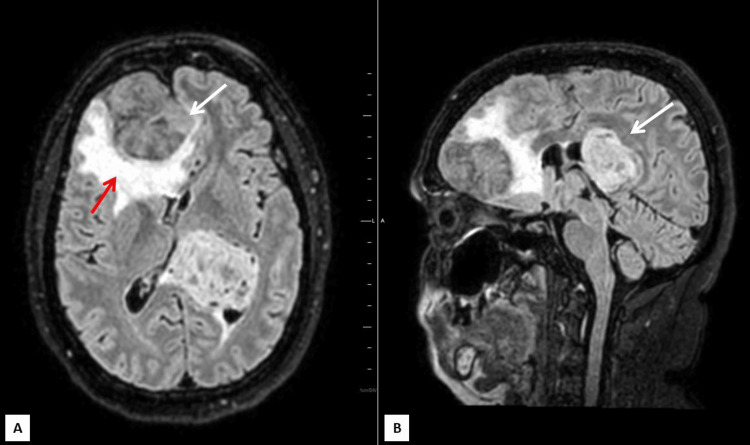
FLAIR magnetic resonance axial (A) and sagittal (B) images show internal heterogeneous signal changes and signs of mass effect in both lesions (white arrows) with perilesional edema seen in the dural-based lesion (red arrow) FLAIR: fluid-attenuated inversion recovery
